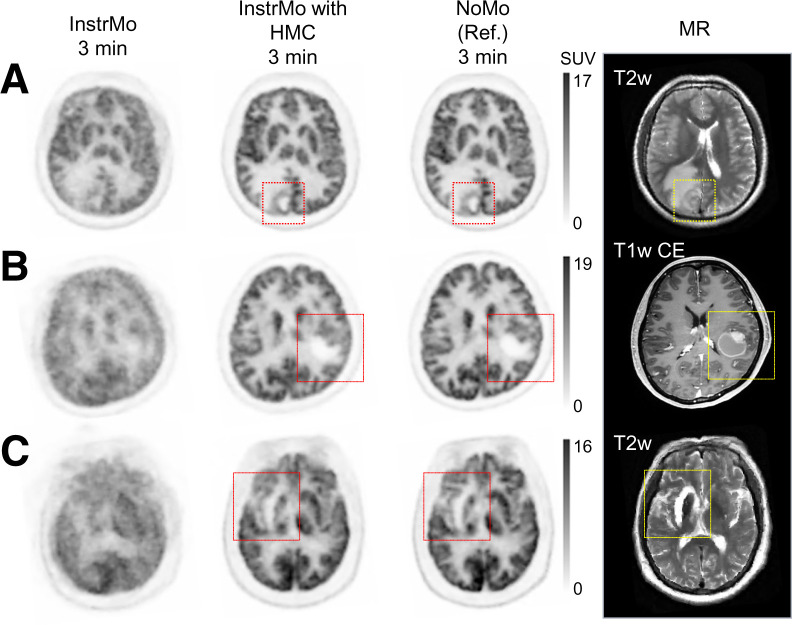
PET and MR images from 3 distinct cases in validation study, comparing InstrMo, HMC, and NoMo. (A) Annular hypermetabolic cerebral syphilitic gumma with surrounding edema in right parietooccipital lobe. (B) Hypermetabolic nodules on PET aligning with nodular wall thickening in cystic-appearing lesion observed on MRI associated with brain metastases from small cell lung cancer. (C) Encephalomalacia and gliosis of right basal ganglia and right temporal lobe and mild *ex vacuo* dilatation of right lateral ventricle in geriatric patient with history of right middle cerebral artery territory infarction. Averaged and maximal HM distance of frontal lobe are 8.5 and 26.3 mm (A), 9.5 and 17.3 mm (B), and 19.0 and 54.2 mm (C), respectively. Injected dose, postinjection time, duration, and body weight are 273.8 MBq, 56.1 min, 3 min, and 69 kg (A); 214.6 MBq, 65.6 min, 3 min, and 56 kg (B); and 270.1 MBq, 67.8 min, 3 min, and 70 kg (C), respectively. T1w CE = T1-weighted contrast-enhanced; T2w = T2-weighted.
